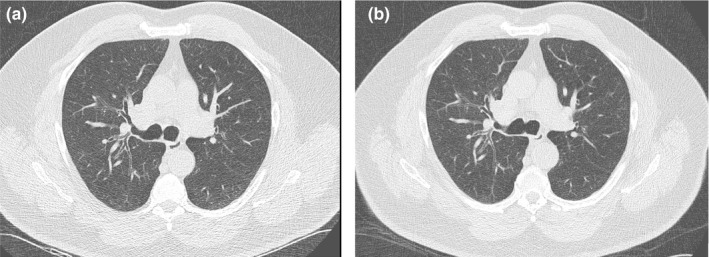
Axial lung images of the largest sized patient who received annual lung cancer screening CT examinations scanned with (a) FTC the first year and (b) TCM the second year. FTC, fixed tube current; TCM, tube current modulation.
